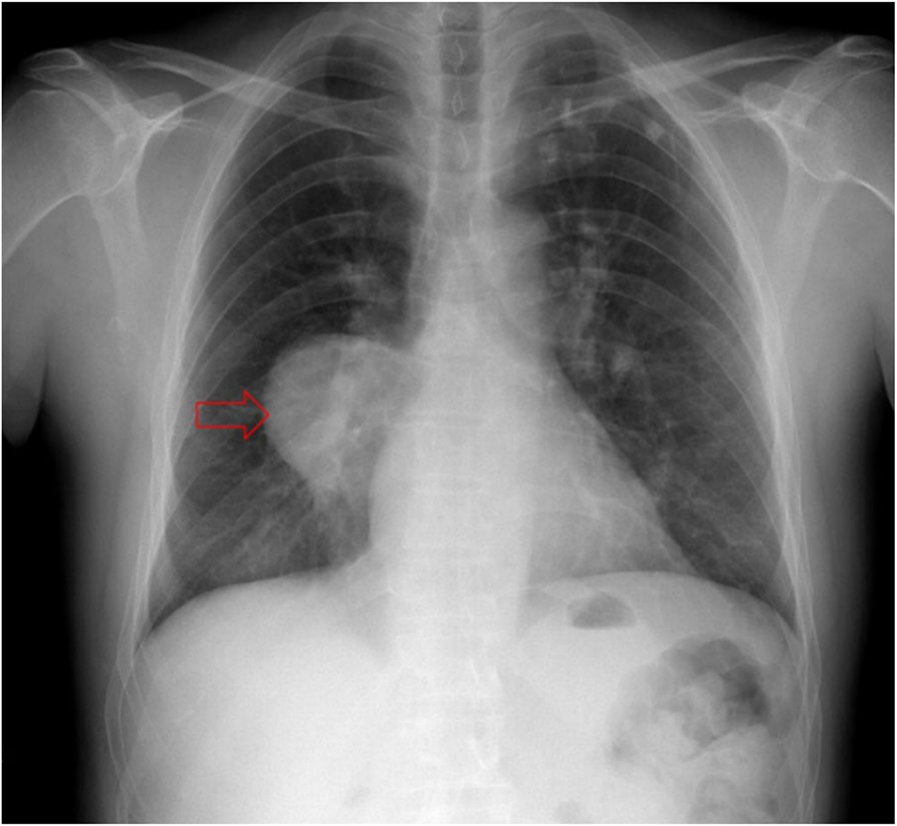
Hydatid cyst has a non-specific appearance on chest radiography
